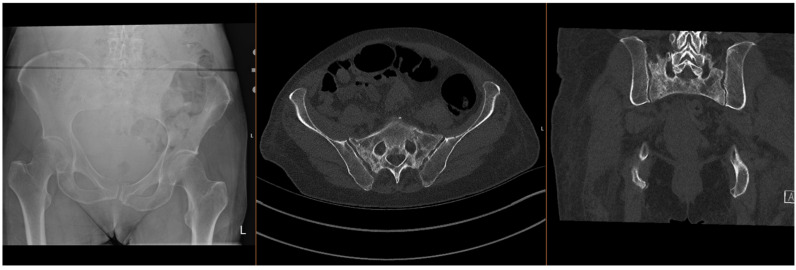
Radiographs and CT images demonstrating common FFP: comminuted bilateral sacral fractures.
